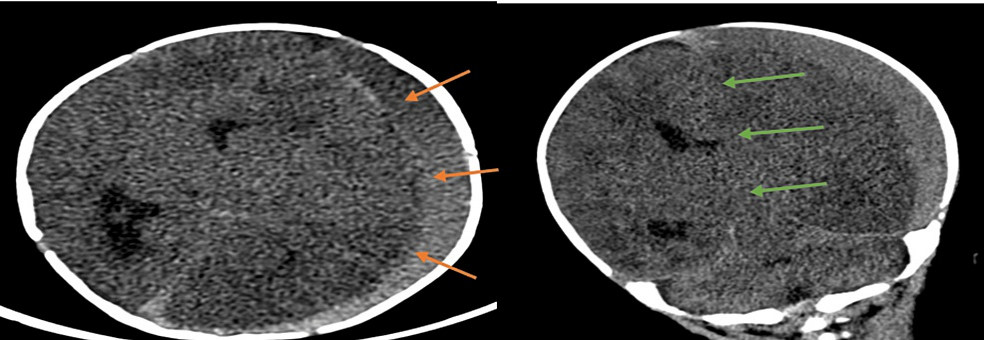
Noncontrast CT brain after initial presentation showing large mixed attenuation subdural hemorrhage involving the left frontal, parietal, temporal and occipital regions (orange arrows) with marked mass effect on the underlying brain parenchyma with 1.4 cm of left-to-right midline shift and asymmetry of the lateral ventricles (green arrows).
